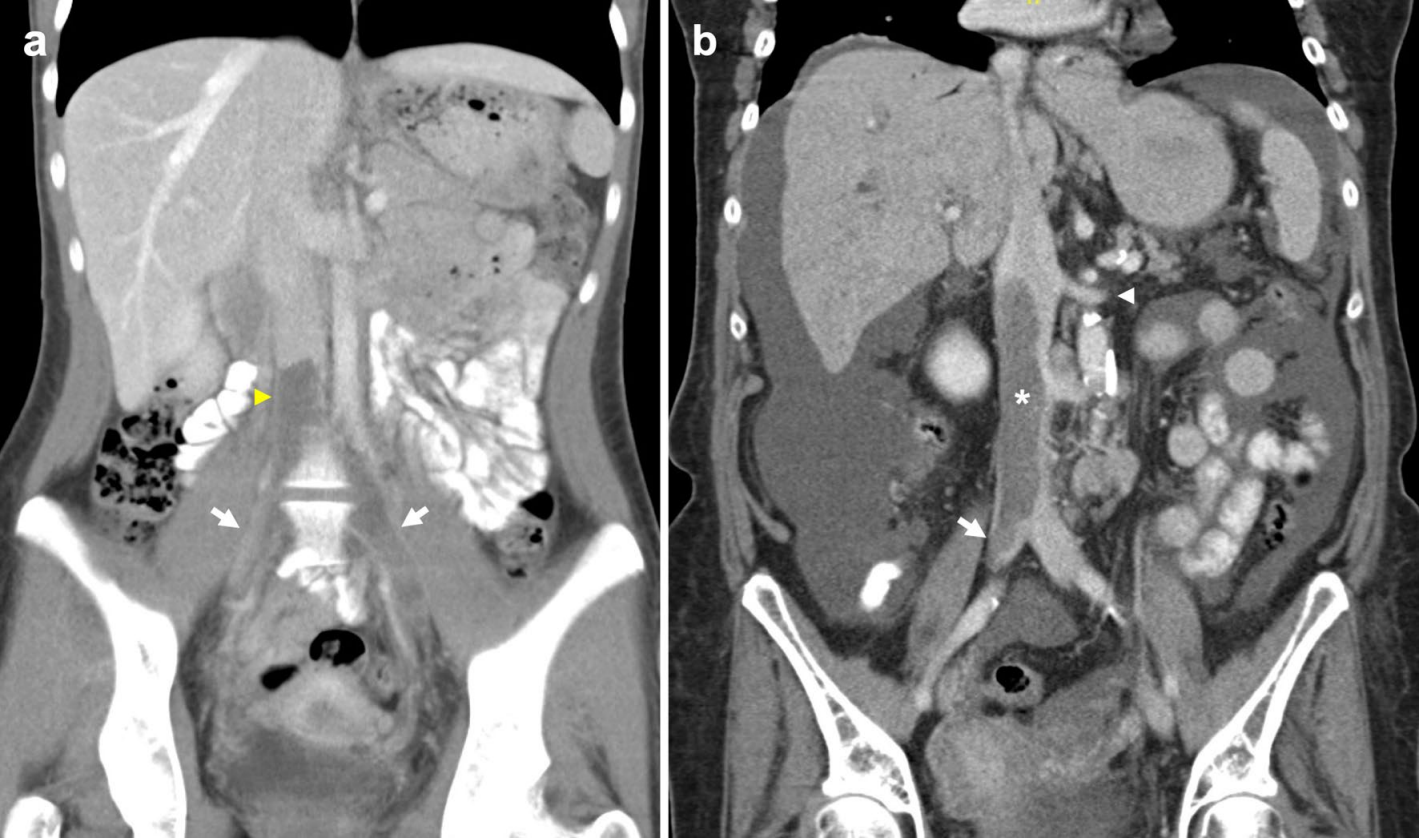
IVC bland thrombosis. a A 20-year-old woman presented with an oedematous left leg and lower back pain in the context of smoking, regular oral contraceptive use and previous DVT. Contrast-enhanced coronal CT shows a non-enhancing filling defect in the infrarenal cava (arrowhead) that propagates into bilateral common iliac veins (arrows). Mild intra-abdominal fat stranding is likely secondary to thrombosis-related oedema. Thrombophilia screen was negative, and there was no evidence of malignancy or venous compression on a CT scan of the chest, abdomen and pelvis. b A 75-year-old woman presented with a left above knee DVT on a background of cholangiocarcinoma with metastases to the liver and left adnexa. Contrast-enhanced coronal CT demonstrates a partially occlusive non-enhancing inferior vena cava thrombus (asterisk) extending superiorly to the level of the left renal vein (arrowhead), and to the right common iliac vein (arrow) caudally
